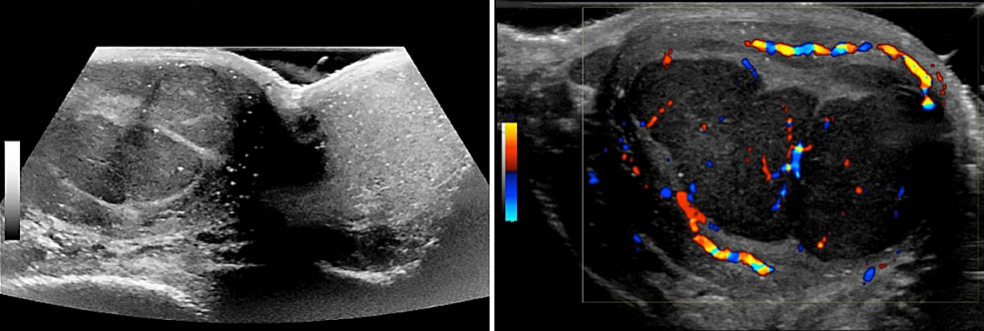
Testicular ultrasound showing multiple echogenic calcifications throughout the right testicle, the largest measuring 3 mm x 3 mm x 4 mm, consistent with testicular microlithiasis. There are no distinct testicular lesions suspected of malignancy.
